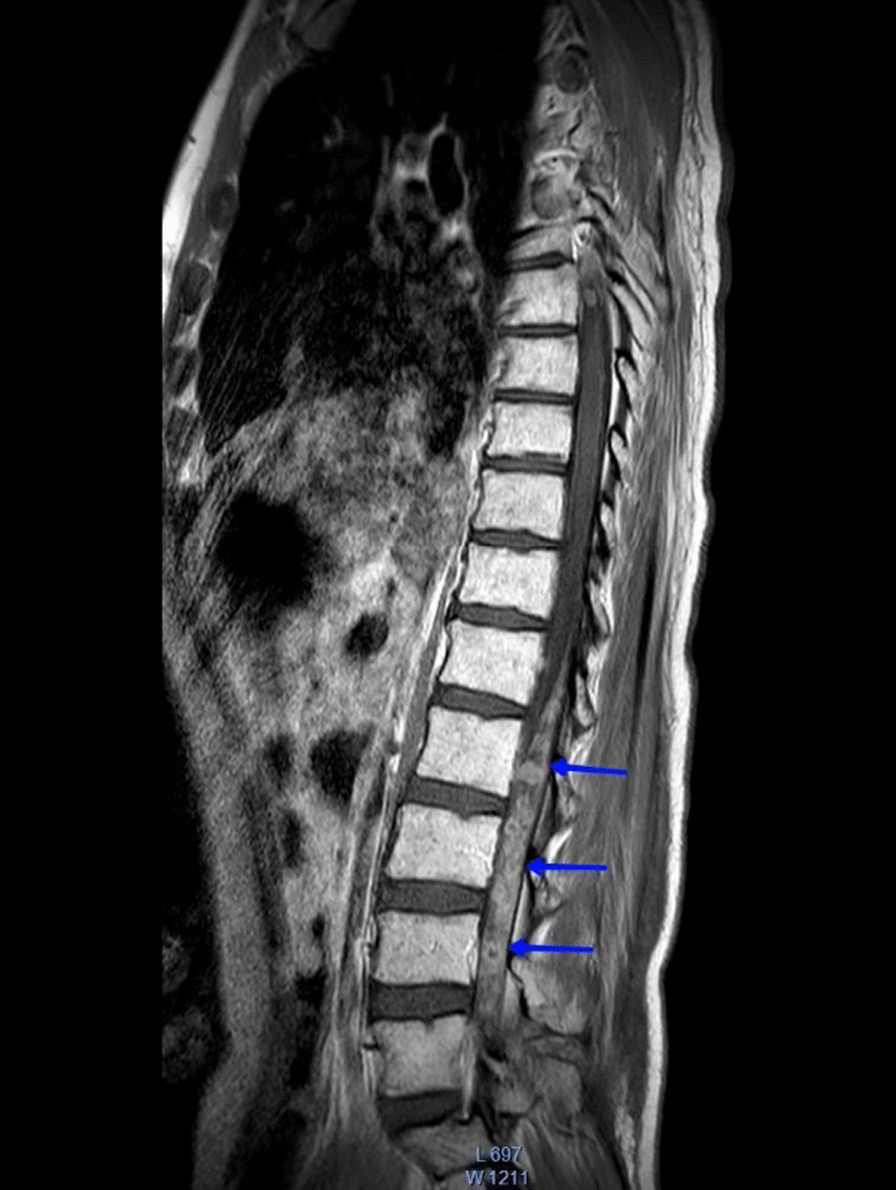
T1-weighted postcontrast MRI in sagittal view showing metastases called drop metastasis (blue arrows) to the medullary cone region
